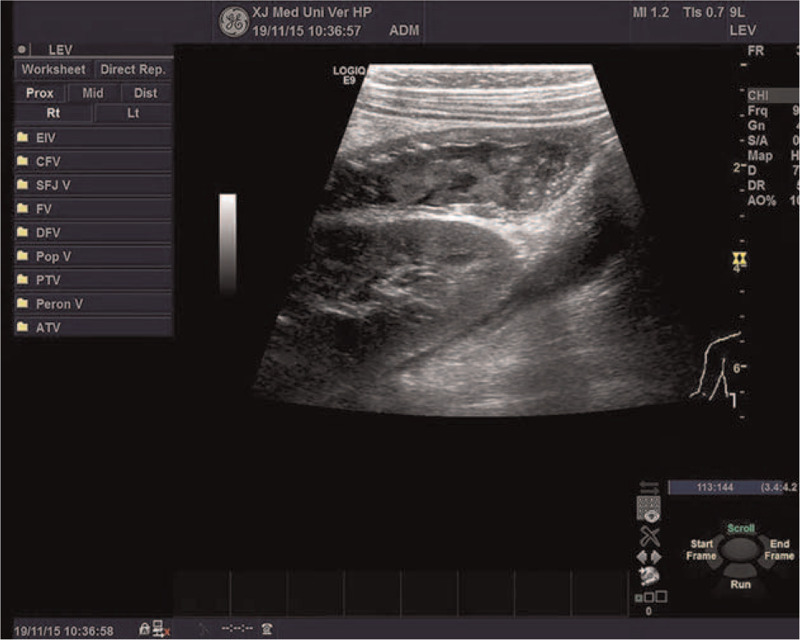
Gastrointestinal ultrasound showed an uneven thickening of the ascending colon and thickening of the surrounding mesentery, about 0.4 cm, suggesting inflammatory changes.
